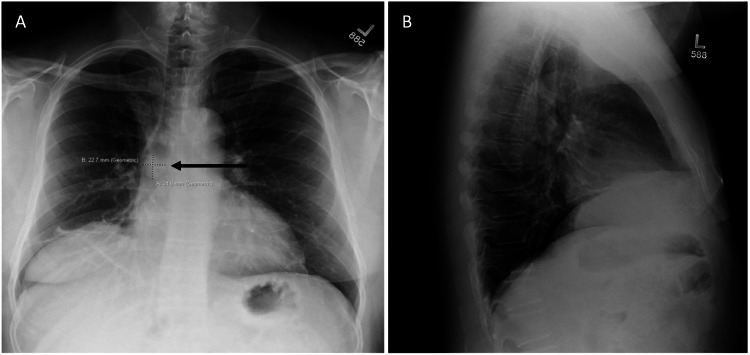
(A) Chest X-ray showing right elevated diaphragm with surrounding atelectasis (black arrow) and the tumor. (B) On the lateral view, the tumor was not clearly evident, so the film was interpreted as not having a tumor.
